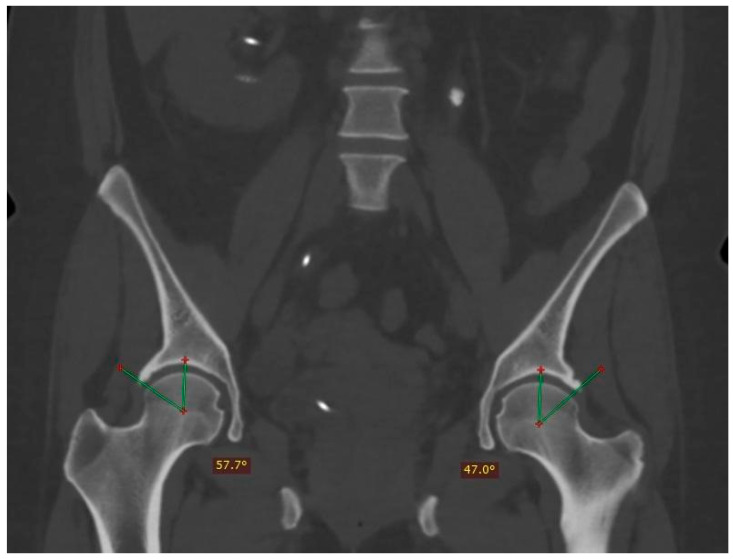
Computed tomography image showing bilateral pincer-type femoroacetabular impingement with coronal reconstruction and window for bone tissues, where acetabular over-coverage was identified with a center-right edge angle of 57.7° and a left angle of 47°. In addition, a stone was observed in the left ureter and a right double J catheter.
